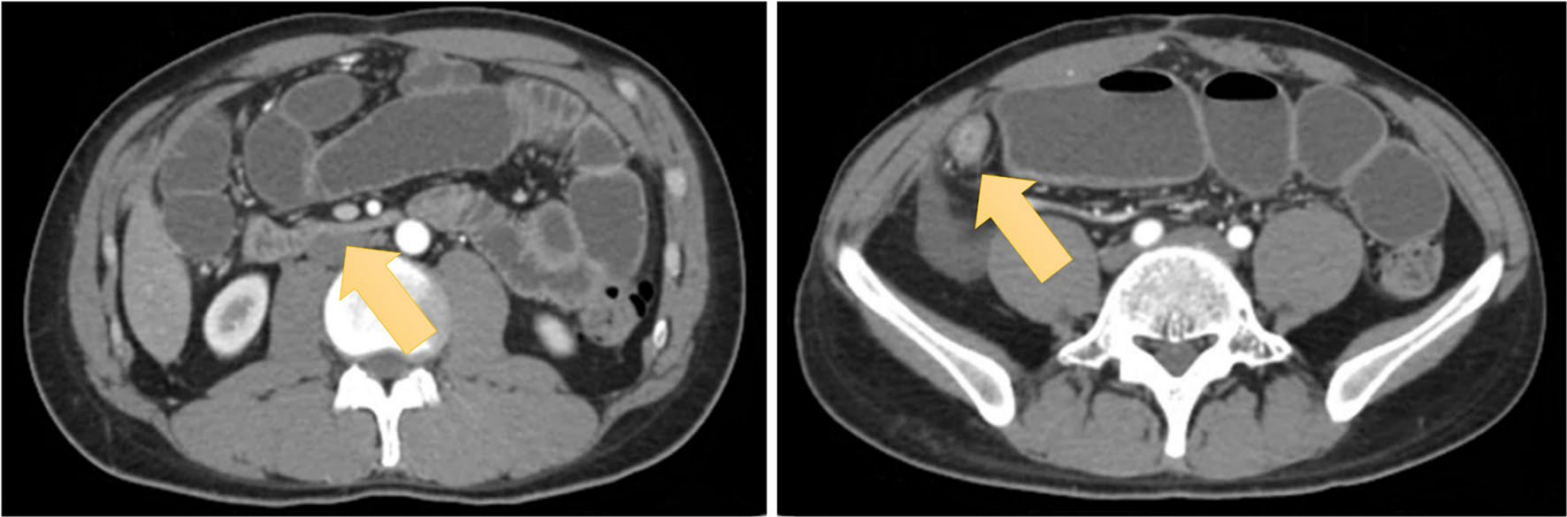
Contrast-enhanced abdominal computed tomography scan shows wall thickening in the ileum with signs of obstruction (arrows)
